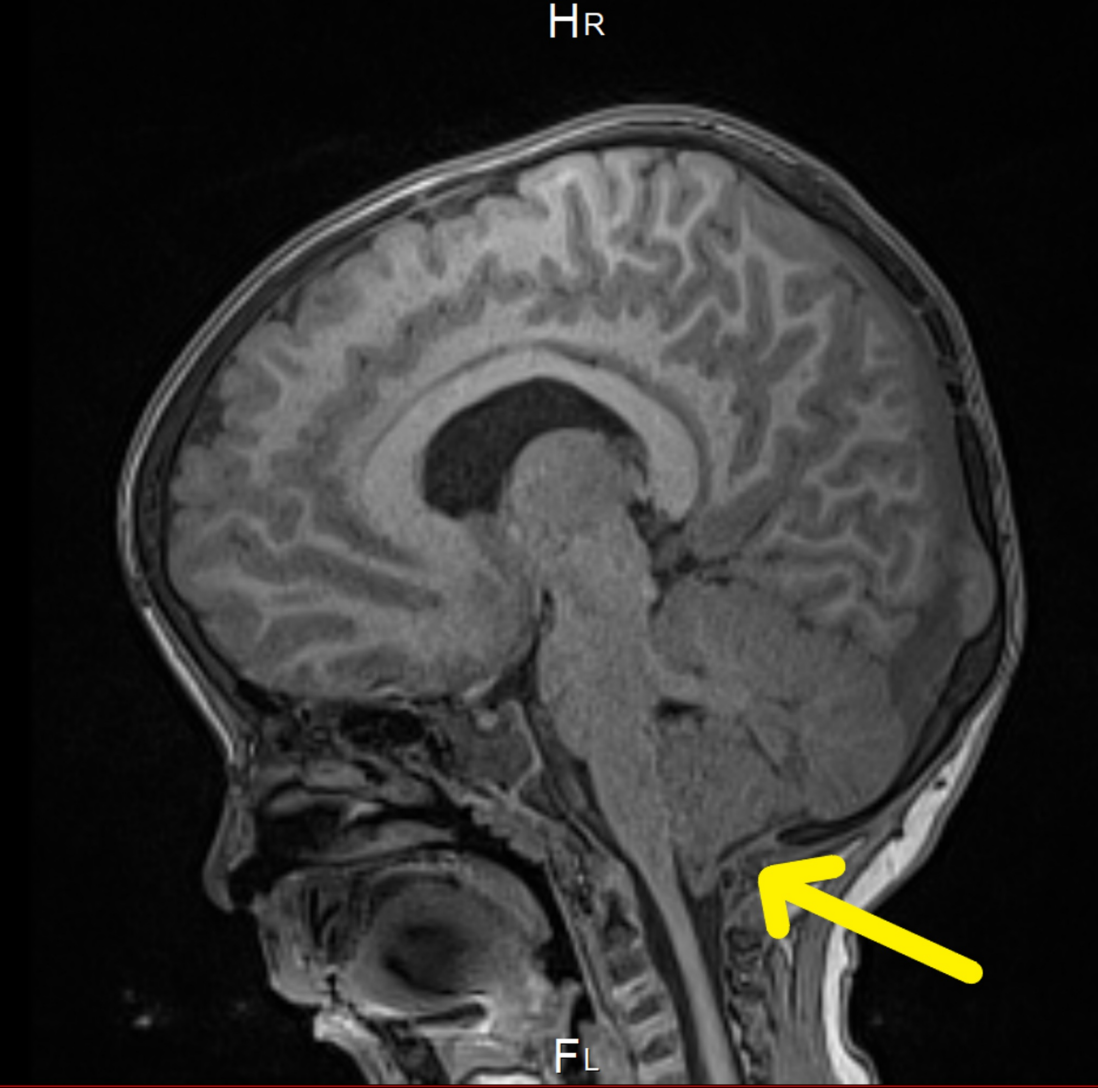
Brain MRI showing cerebellar tonsil ectopia with crowding of the posterior fossa
